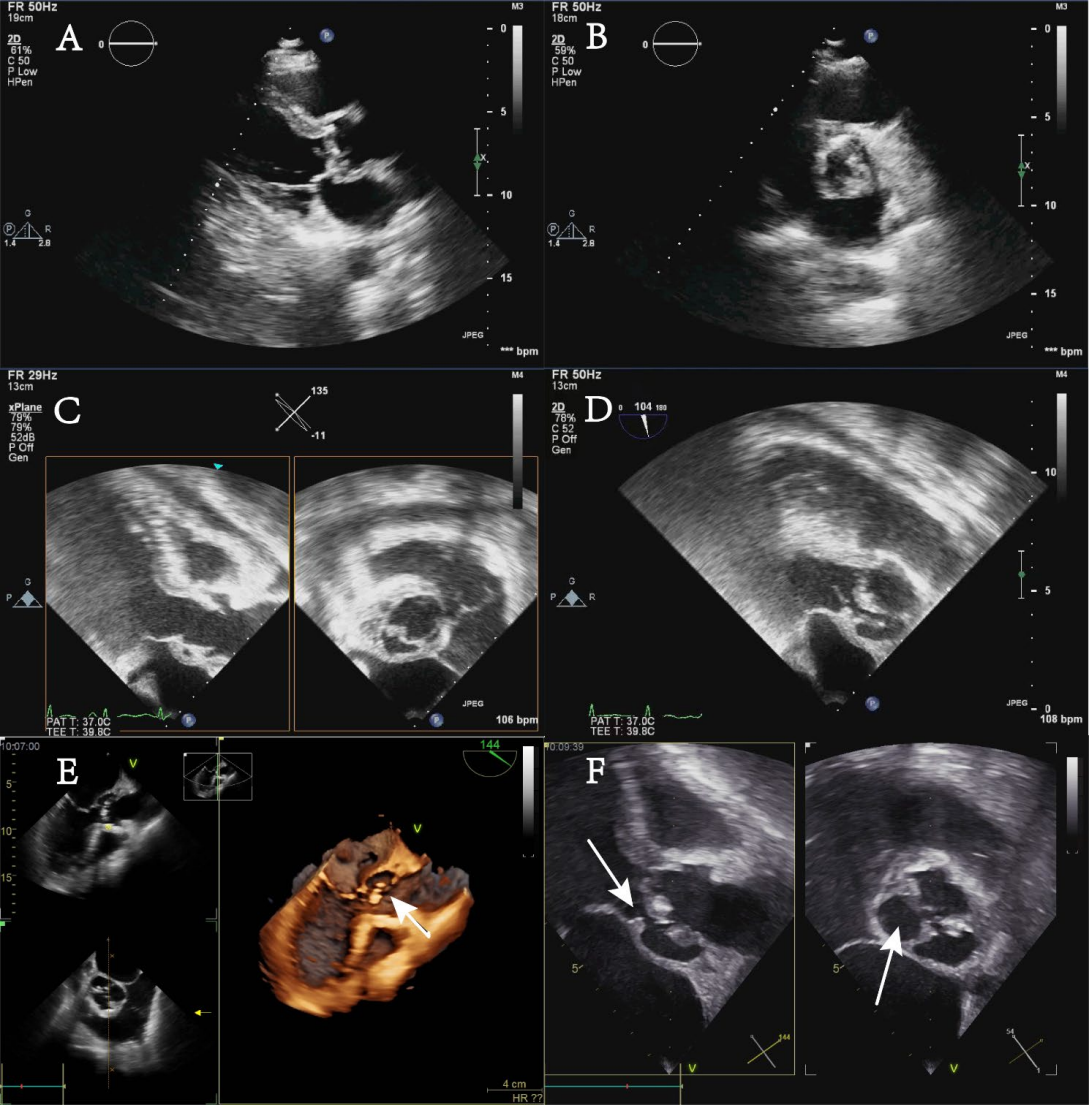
Echocardiographic images at different times. (A) Parasternal long axis section of TTE, a 16.4 mm*7.8 mm vegetation can be seen on the right posterior aortic valve. (B) Parasternal Aortic Short Axis Right Ventricular Outflow Tract Section of TTE, the aortic valve is calcified bicuspid combined and a possible paravalvular abscess (increased thickening in the right posterior sinus wall of the aorta). (C and D) The bicuspid aortic valve is arranged in front of and behind, with rough leaflets and multiple mixed echo mass shadows attached to the edge of the leaflet. The maximum echo mass is about 12.4 mm * 6.2 mm in the anterior valve, which is smaller than that seen in TTE 10 days ago. (E and F) Half a month later since last TEE, a large area of abscess around the aorta leads to a pseudoaneurysm of the sinus of Valsalva, which flows into and out of the left ventricle
